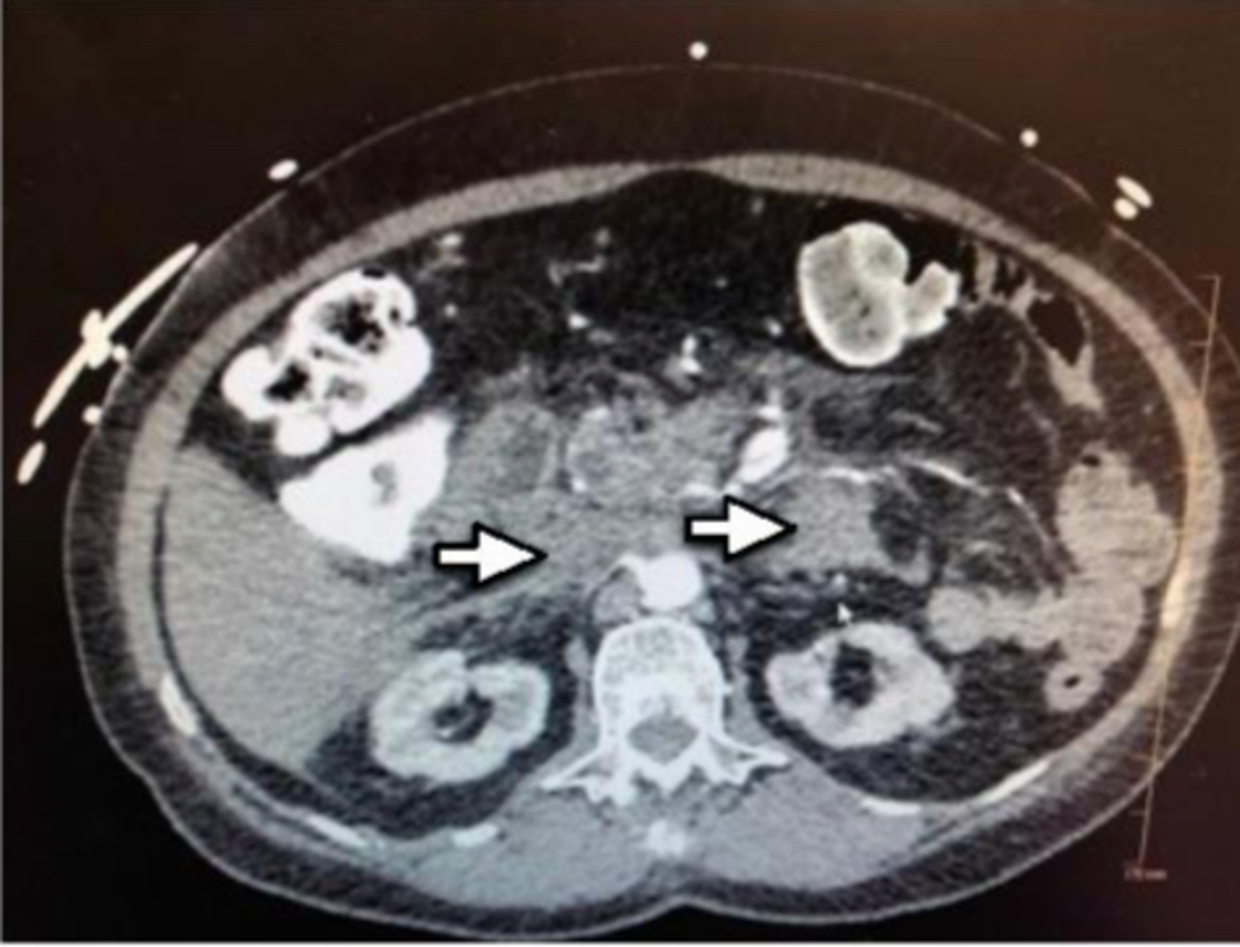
CT of the abdomen showing a diffuse hemorrhage in the peritoneum as indicated by the arrow.
